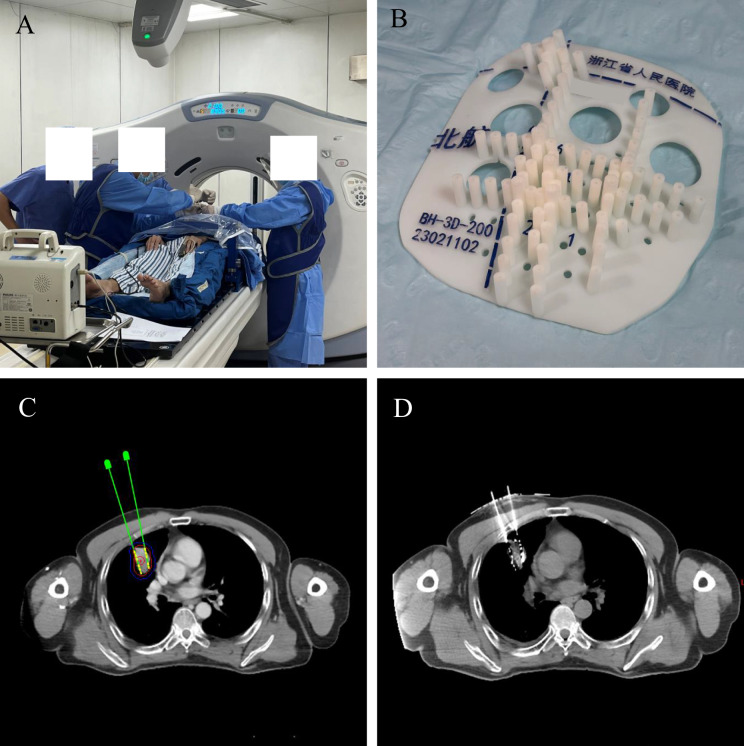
(A–D) The clinical operation of 3D-PNCT assisted CT-guided RISI.
